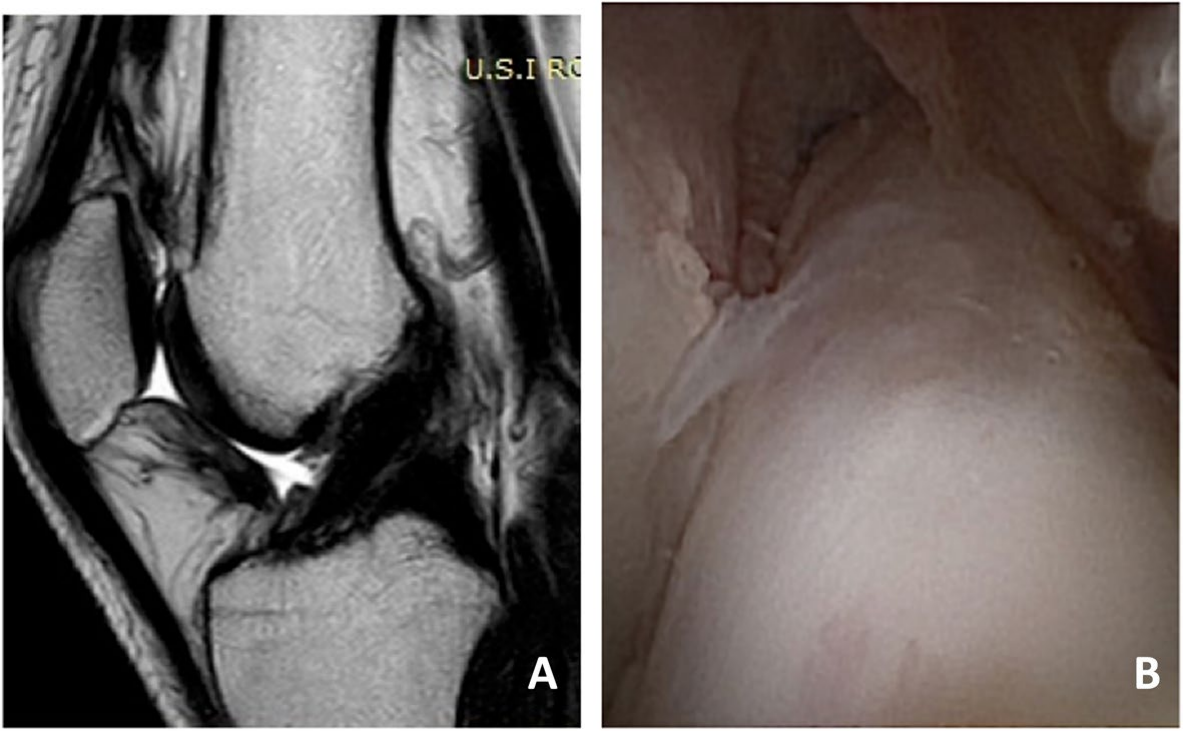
ACL repair. A Sagittal T2-TSE MRI post-operative 6 months after ACL repair (Howell I); B In-office needle arthroscopic view of the repaired ACL of the same patient showing type A healing
